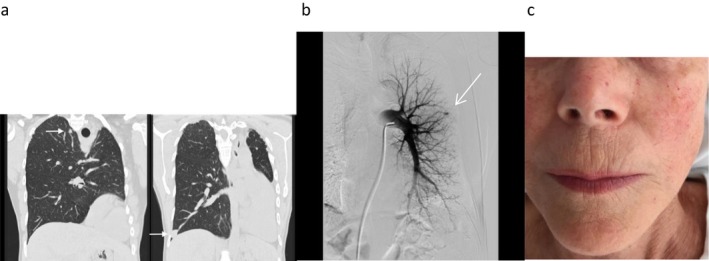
(a) CT scan of the lung of the proband. Two residual pulmonary AVMs (arrows), and only one right lung (left‐sided pneumonectomy due to severe pulmonary AVM at age 10). (b) Pulmonary angiography of the mother, with one large PAVM just prior to embolization. (c) The face of the mother at age 76, with few but typical telangiectatic lesions
